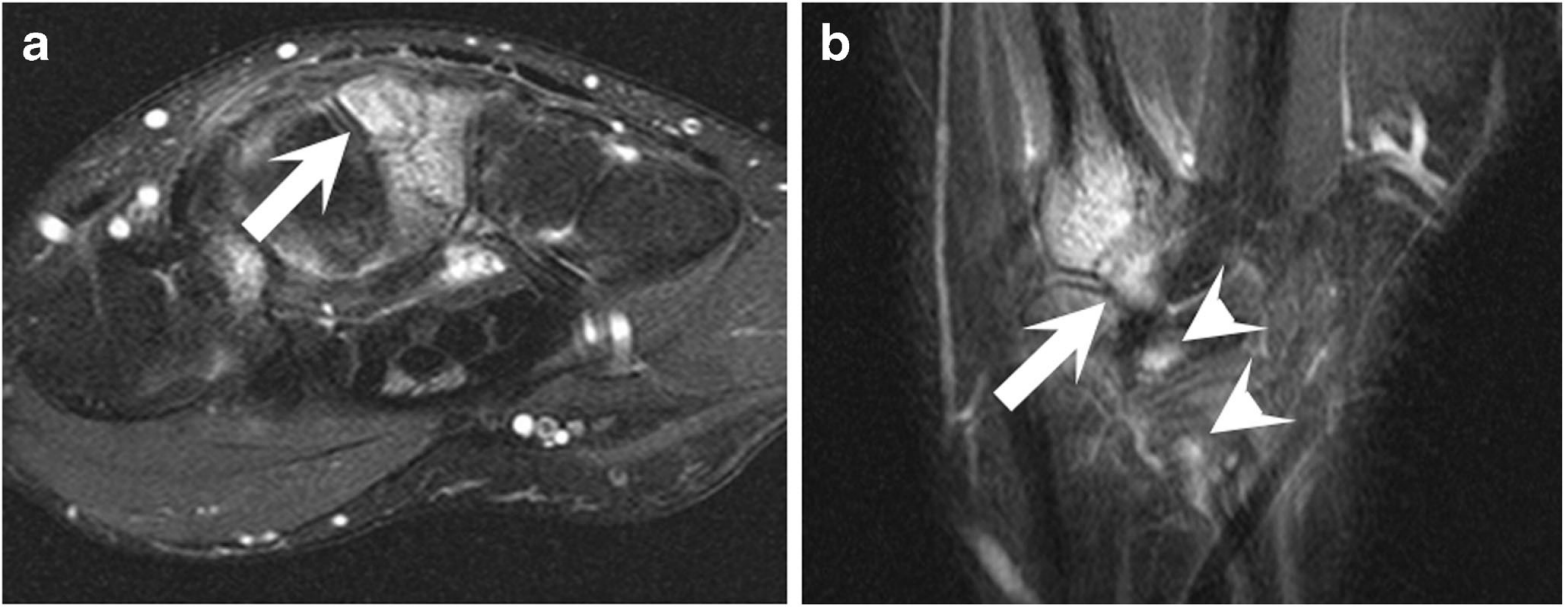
a Axial proton-density-weighted fat-suppressed image and b a coronal proton-density-weighted fat-suppressed image from a wrist MRI performed on a 38-year-old man after hyperflexion trauma in a fall shows a fracture at a partially fused carpal boss, with extensive bone marrow edema (*white arrows*) and extensive tendinous edema in the distal extensor carpi radialis brevis tendon (*white arrowheads*)
